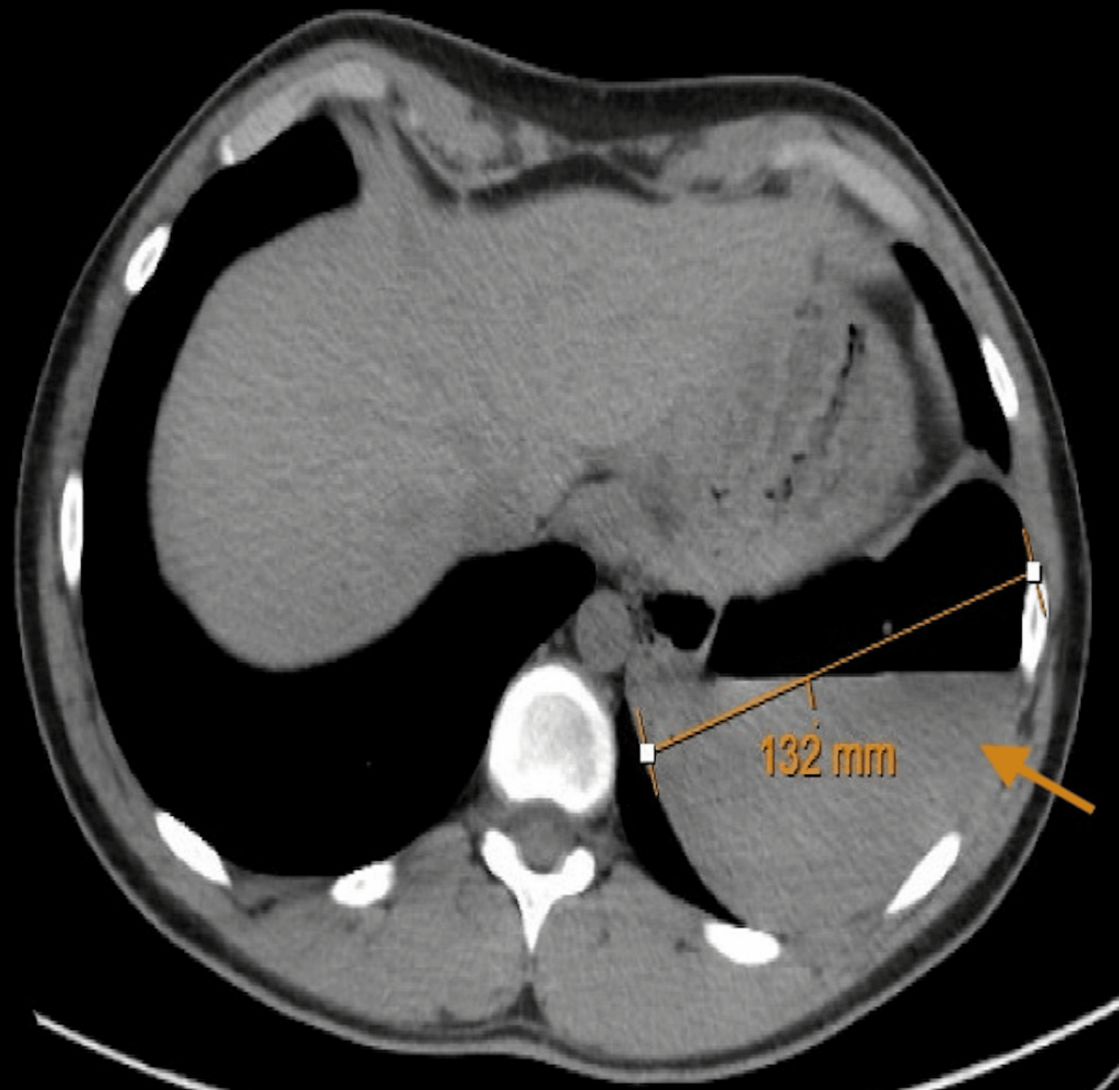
Axial CT scan of the chest showing a large 13.2 cm thin-walled cavitary lesion with an air-fluid level in the left lower lobe, as indicated by the arrow
